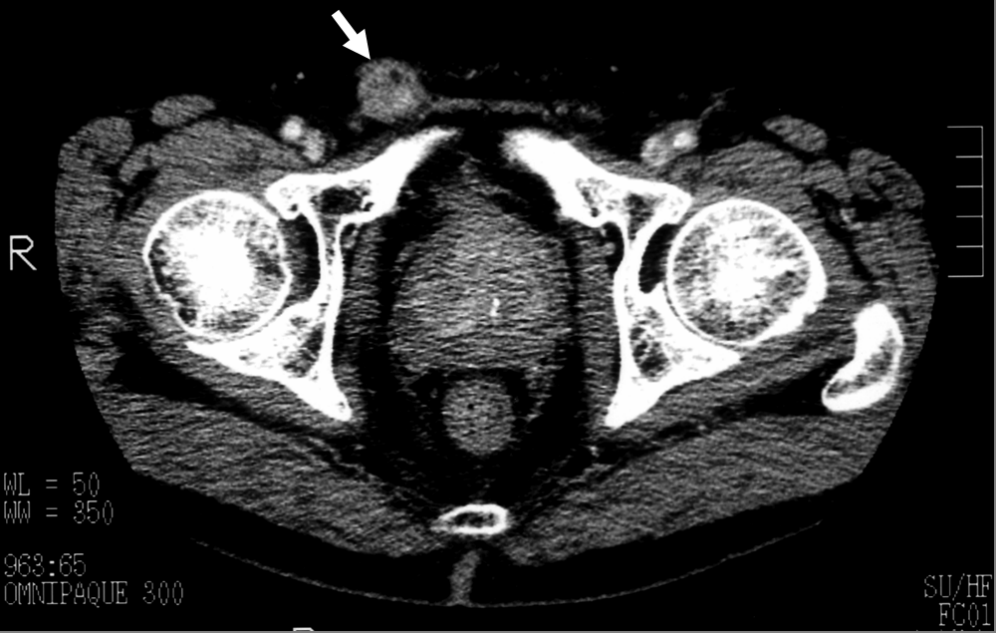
Contrast computed tomography scan image showing the inguinal tumor with heterogeneous enhancement (arrow).
